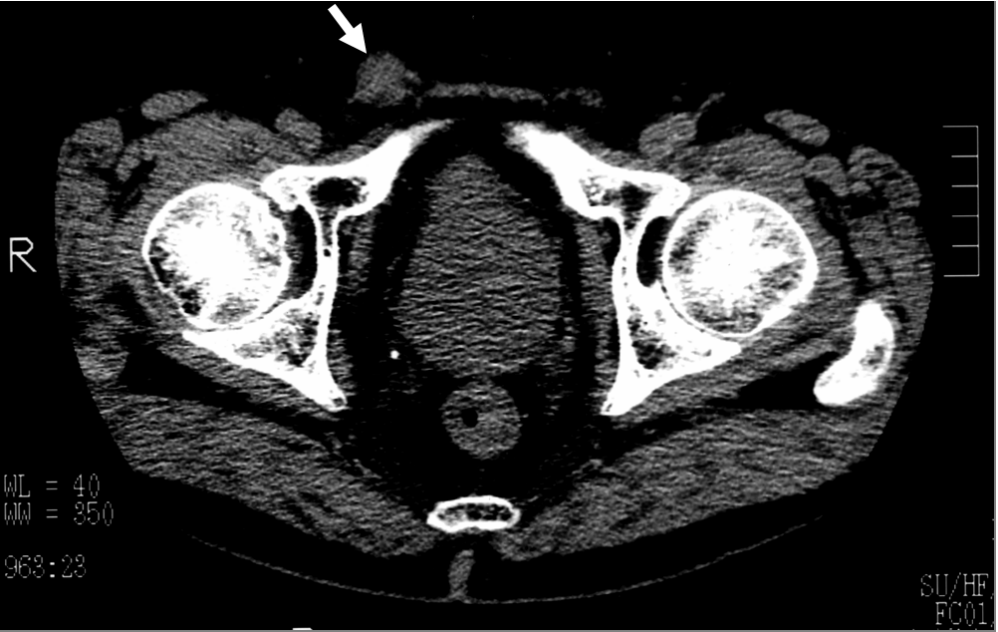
Plain computed tomography image showing a soft tissue density mass (arrow) within the right inguinal canal. The mass had no connection with intra-abdominal organs.
